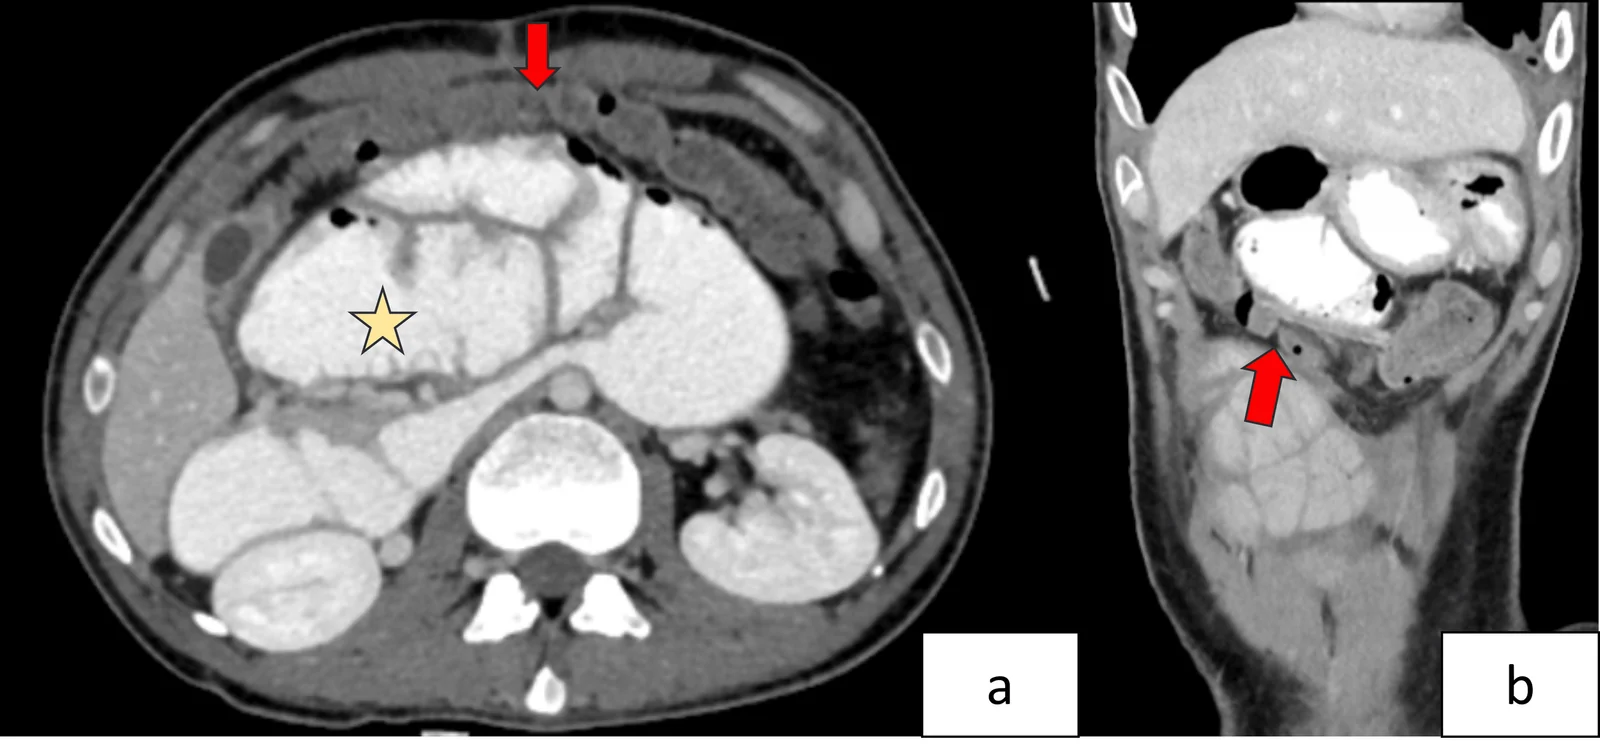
CECT images demonstrating transmesocolic internal hernia 3a Axial and 3b sagittal CECT images demonstrate dilated small bowel loops containing positive oral contrast within (yellow star) herniating through a transverse mesocolic defect. The herniated bowel loops are located posterior to the stomach and compress the transverse colon anteroinferiorly (red arrows), consistent with a transmesocolic internal hernia. CECT: contrast-enhanced computed tomography
